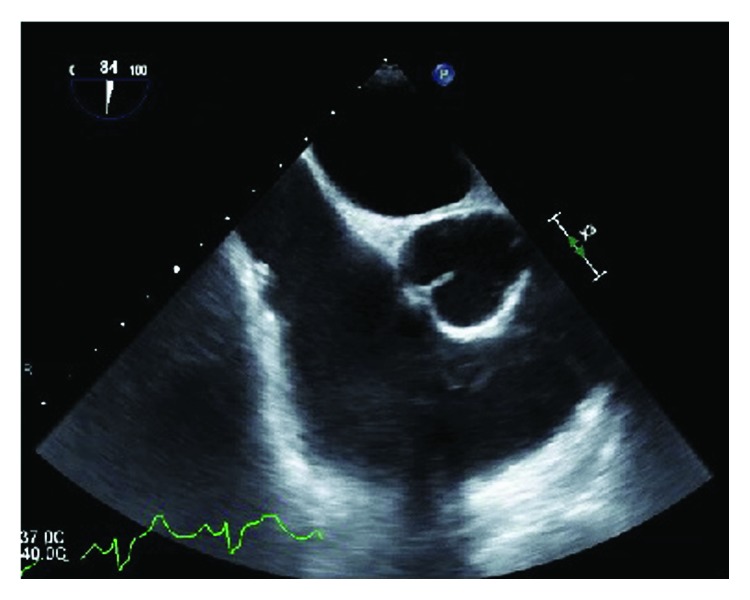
Midesophageal short axis view obtained on transesophageal echocardiogram performed on hospital day 3 with a focus on the right atrium, tricuspid valve, and RVOT. There was no clear, identifiable source of infection on this initial evaluation.
